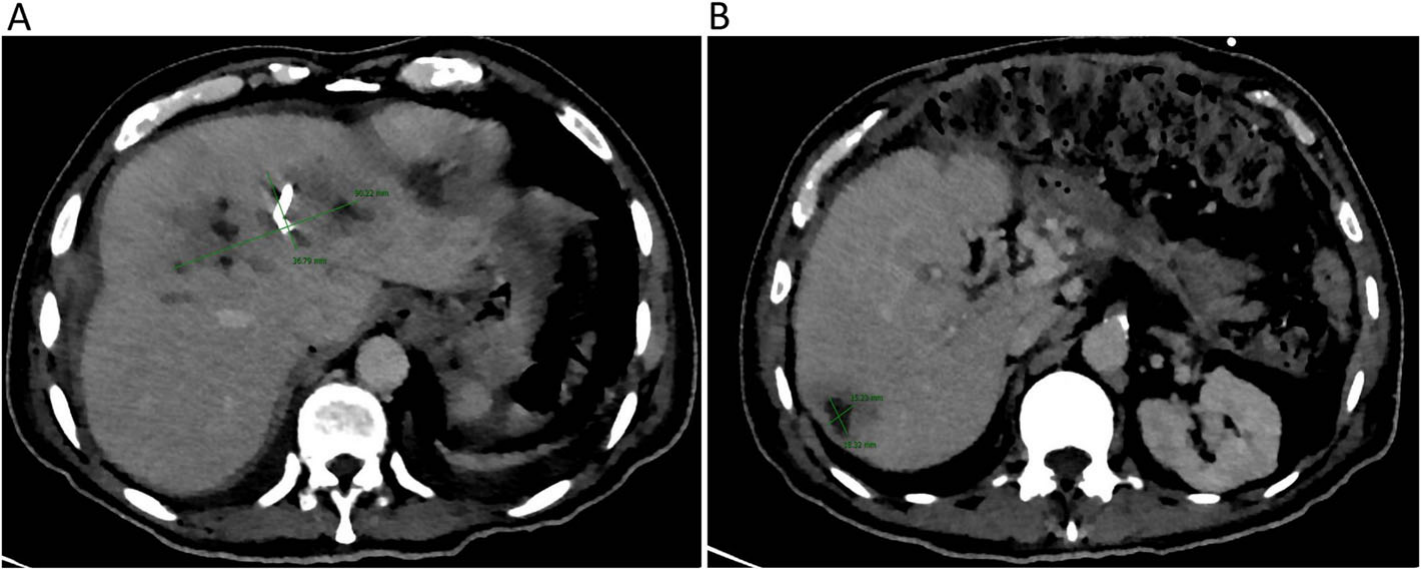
CT scan was taken 10 days after the CT-guided percutaneous drainage was performed. (A) The distal portion of a 10 Fr Dawson-Müller multipurpose drainage catheter can be seen in the interior of the abscess localized on liver segment VIII. Gas is present in the interior of the abscess because of the multipurpose drainage catheter insertion. Note the smaller size of the abscess (12.1 cm × 4.2 cm) compared with the first CT scan (14.5 cm × 5.4 cm). (B) The abscess on liver segment VI also decreased in size with 10 days of antibiotic therapy, measuring 1.8 cm × 1.5 cm (previously 3.1 cm × 2.7 cm)
